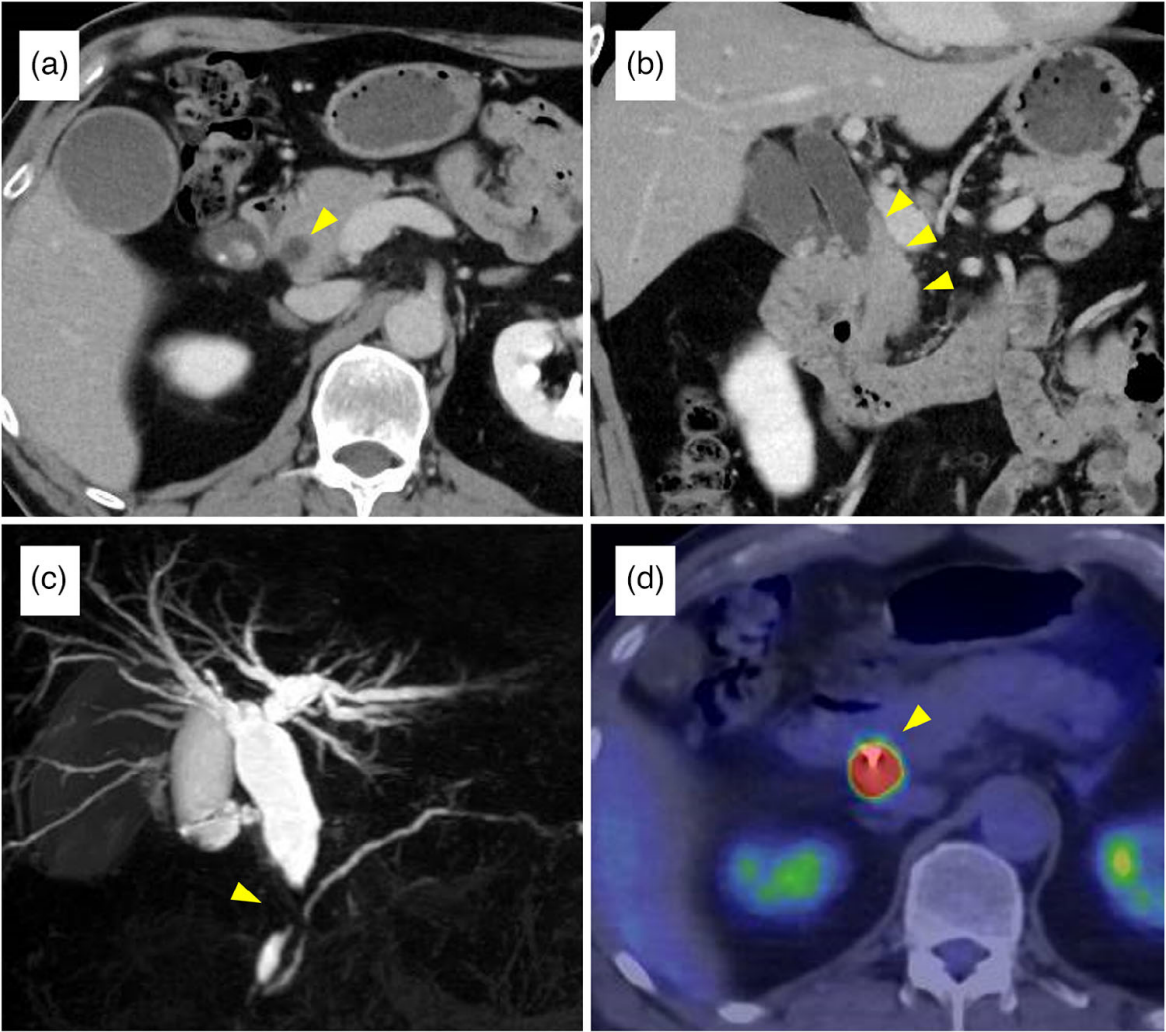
Radiological findings of the bile duct lesion: (a, b) Axial and coronal contrast‐enhanced computed tomography images showing irregular thickening of the distal bile duct wall. (c) Magnetic resonance cholangiopancreatography reveals stricture of the distal bile duct with dilatation of the proximal bile duct. (d) Positron emission‐computed tomography reveals abnormal 18‐fluorodeoxyglucose uptake at the site of the bile duct stricture. The maximum standardized uptake value is 32.29.
